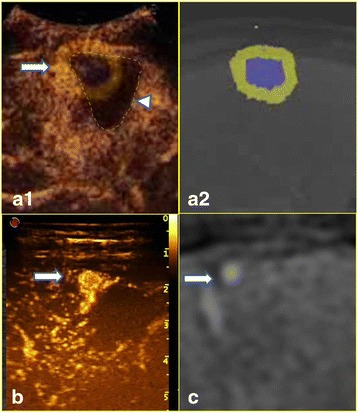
a1 and a2 show the CEUS-CT fused image. a1 shows the overlapped image of CEUS and CT. The HCC lesion and 5-mm AM are outlined in *blue* and *yellow*, respectively. The ablative area in CEUS was anechoic and is outlined with a dotted line (*arrowhead*). In a1, the ablative area just covers the tumor, and part of the AM is not covered by the ablative area (*arrow*). Thus, the CEUS-CT fused image shows that this HCC lesion has an inadequate AM at the site indicated by the *arrow*. In the CT image in a2, the HCC lesion and 5-mm AM are outlined in *blue* and *yellow*, respectively. One month after ablation, the CT revealed complete ablation of the lesion, and the serum AFP fell to a normal level. However, 9 months after ablation, the serum AFP again increased. LTP was shown by CEUS (b) and CT (c) at the same site with an inadequate AM as in a1 (*arrow*)
